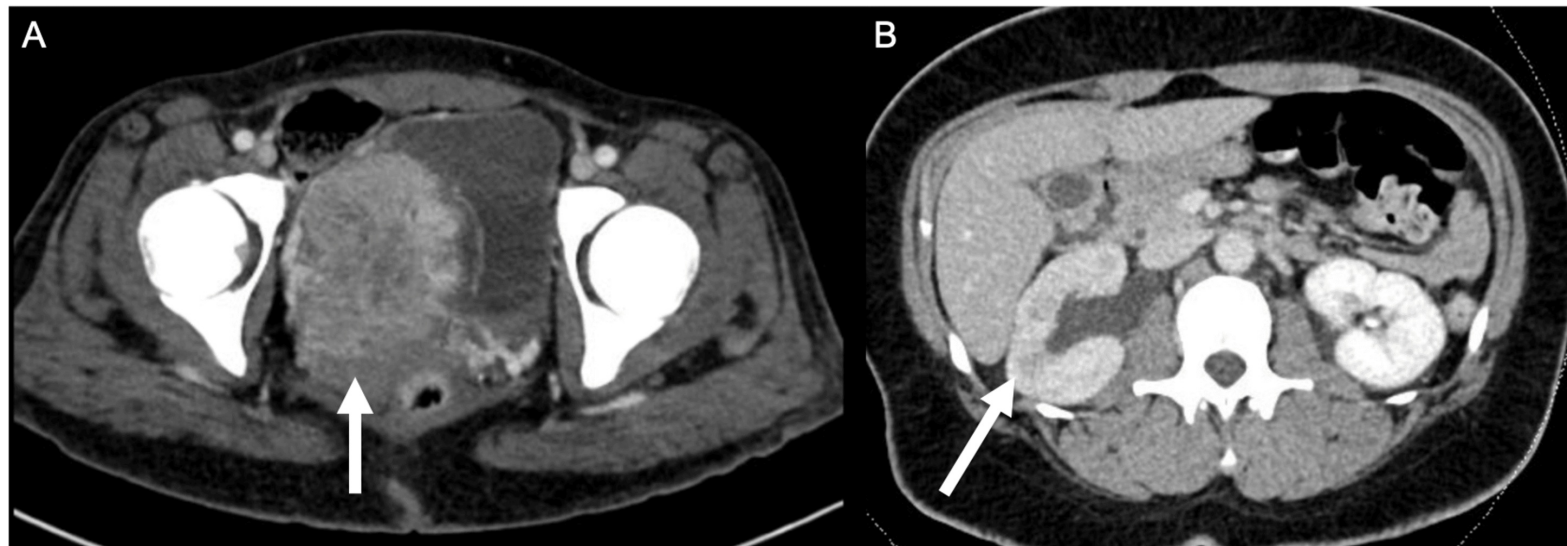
A) Axial view of CT scan showing large volume bladder tumor (white arrow). B) Axial view of CT scan showing right hydronephrosis with delayed nephrogram (white arrow).
